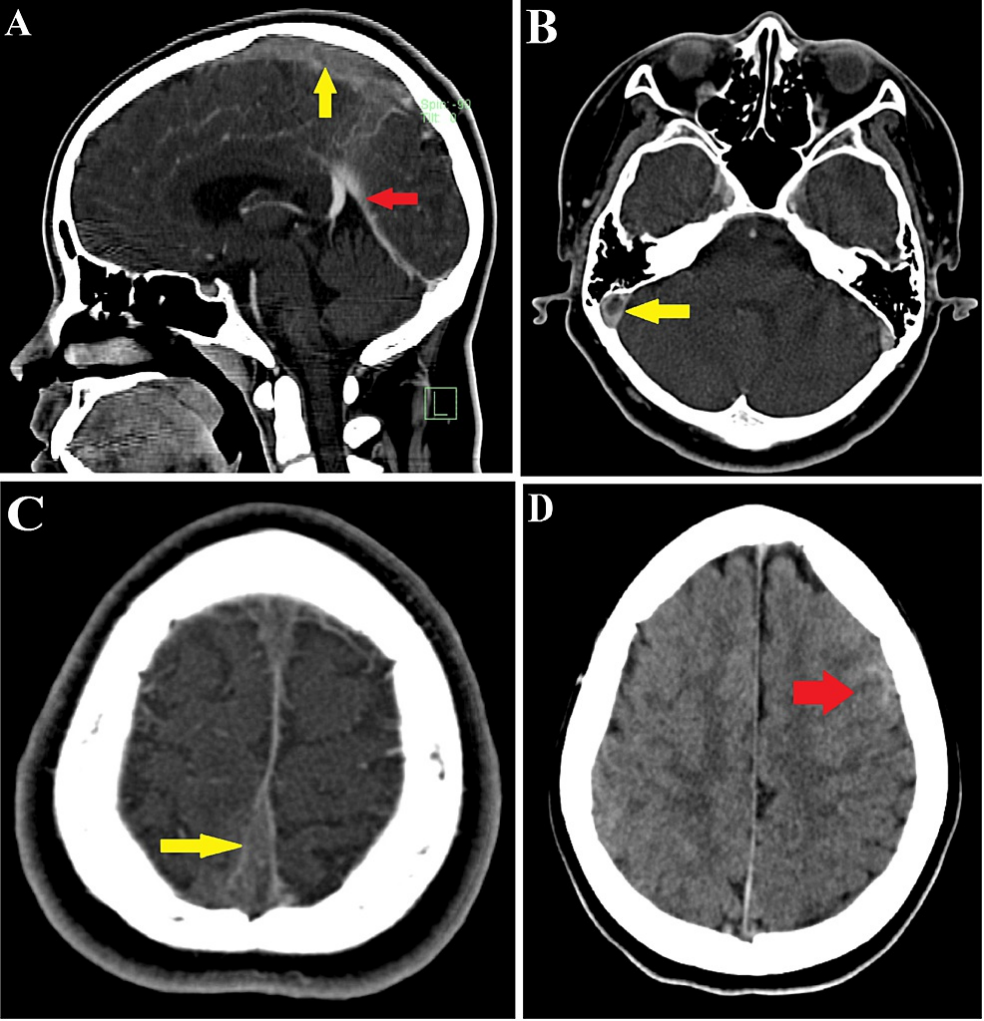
Computed tomography (CT) of the brain. (A) Thrombosis of the superior sagittal sinus (yellow arrow) and cerebral vessel with normal contrast enhancement (red arrow). (B) Thrombosis of the right transverse sinus (yellow arrow). (C) Thrombosis of the superior sagittal sinus (yellow arrow). (D) Intracranial hemorrhage in the left frontal and parietal lobe (red arrow).
